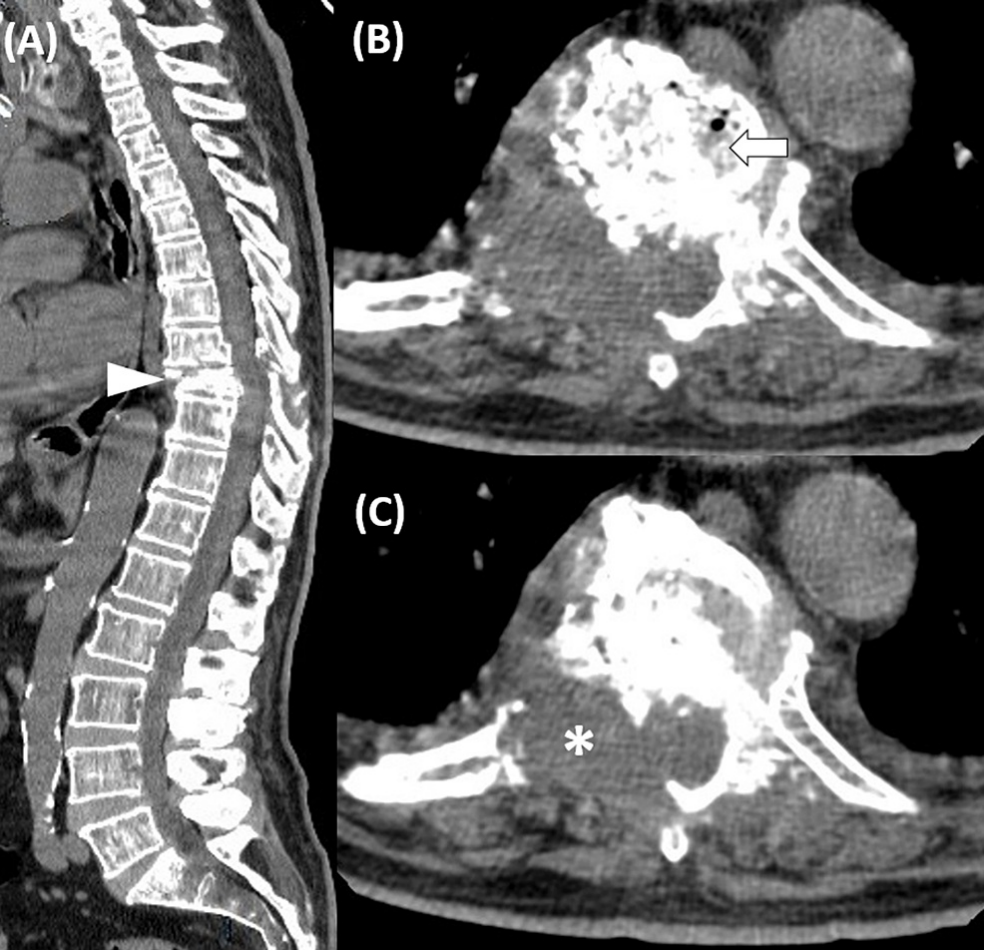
Contrast-enhanced computed tomography (A) Sagittal plane: fracture and collapse of the ninth dorsal vertebra body (arrowhead). (B) Horizontal plane: lytic lesion of the vertebral body (arrow). (C) Horizontal plane: soft tissue formation extended to the right paravertebral space as well as to the anterior epidural space (asterisk).
